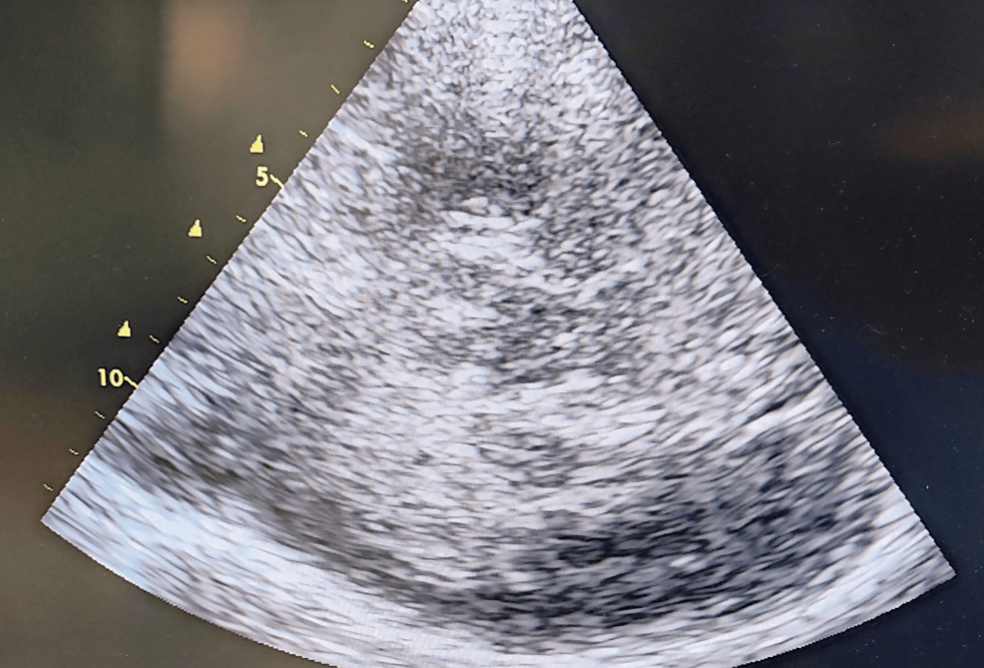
Hyperechoic image on cerebral ultrasound in relation to an intraparenchymal hematoma.
